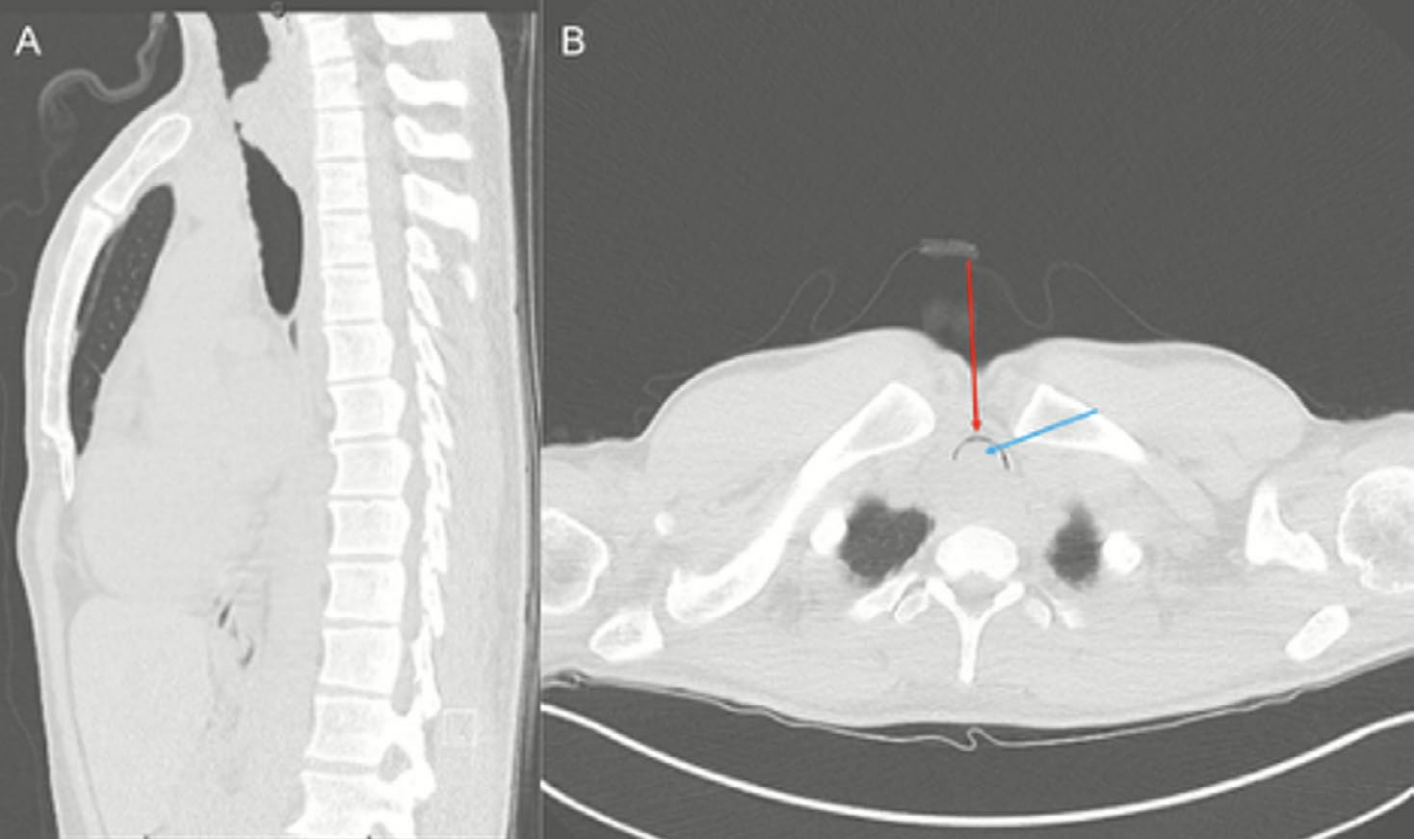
CT chest. (A) CT chest in the sagittal view shows near complete loss of tracheal lumen within the thorax. (B) Axial view of CT chest shows a sliver of patent airway in the anterior distal trachea reflected by a crescent of air (red arrow) and what is an obstructive soft tissue density occupying the remainder of the tracheal lumen (blue arrow).
